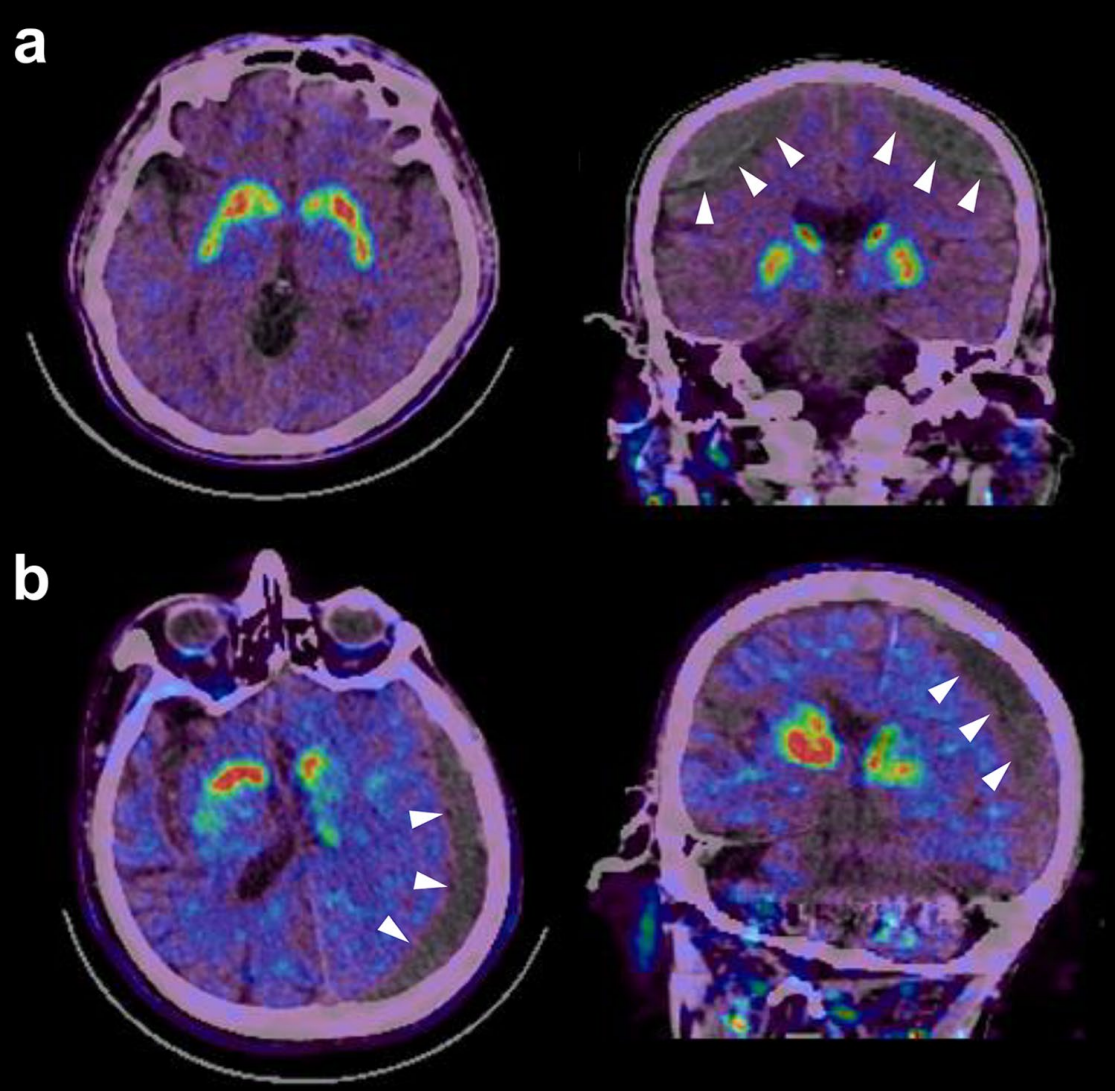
(a) Representative cases with subdural hematoma detected by [^18^F]FP-CIT PET/CT performed for initial evaluation of parkinsonism. (a) Transaxial and coronal [^18^F]FP-CIT PET/CT fusion images of a 73-years old man who complained of gait disturbance and bradykinesia after a traffic accident one month ago show normal striatal uptake and subdural hematomas (arrowheads) in the bilateral frontal convexity. (b) Transaxial and coronal [^18^F]FP-CIT PET/CT fusion images of a 74-years old man who complained of frequent falls and gait disturbance show reduced striatal uptake and also subdural hematoma (arrowheads) in the left parieto-temporal convexity
